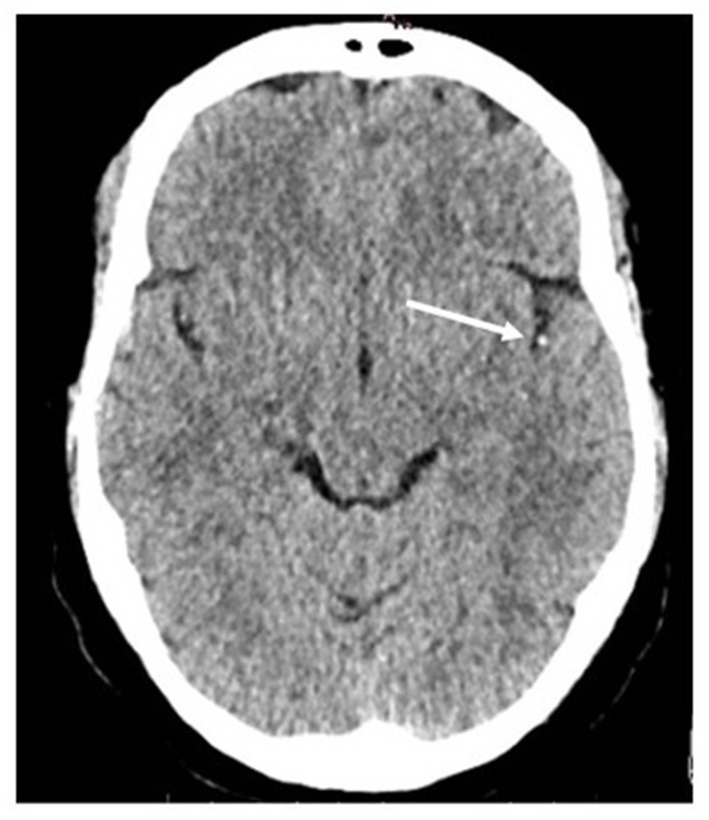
Example of hyperdense artery sign (HAS) in M2 segment occlusion on the left (arrow), in non-contrast CT scan. The extracted clot was a RBCs-rich clot.
